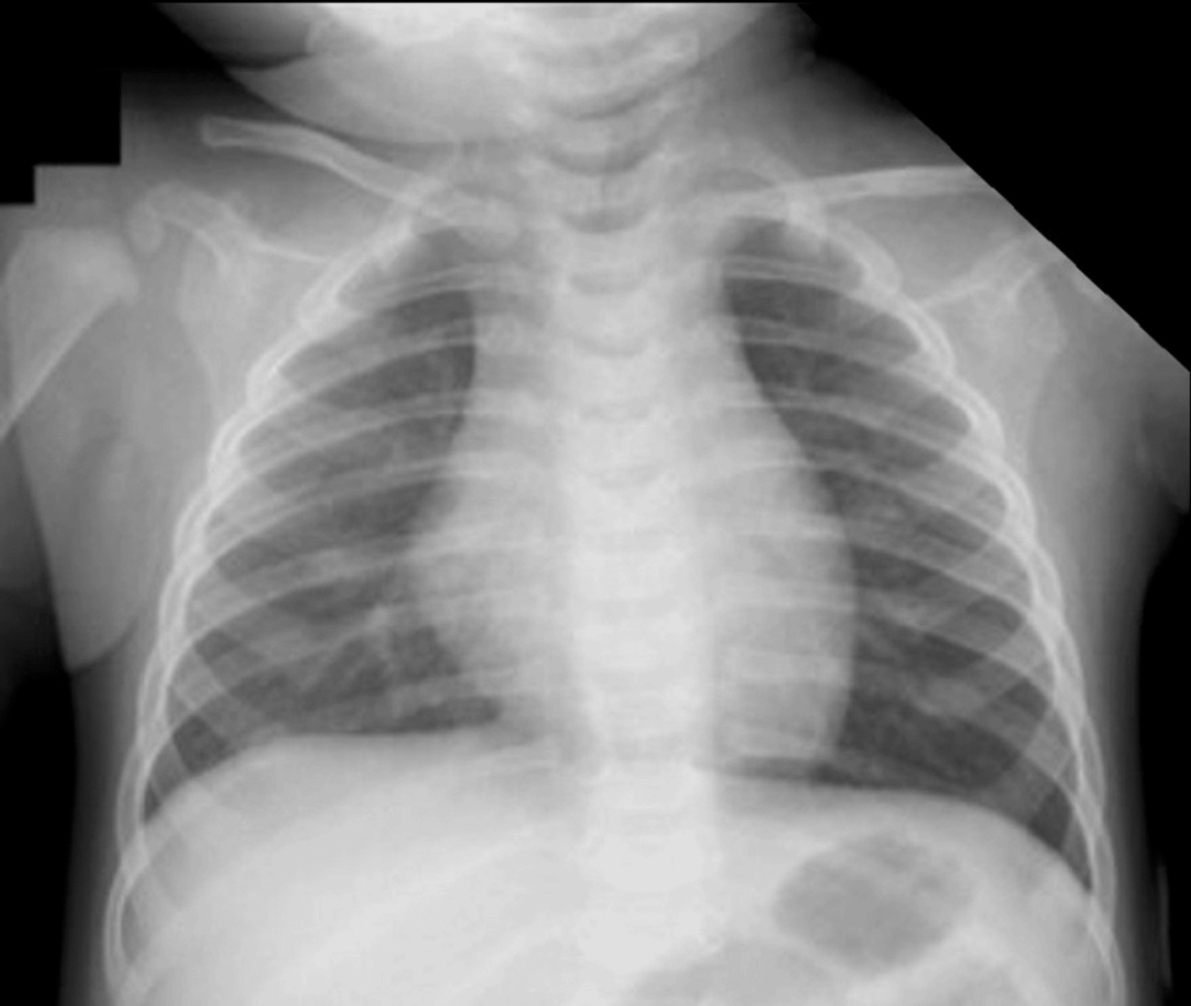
Preoperative chest X-ray The preoperative chest X-ray shows no abnormal findings in the lung field and mediastinum.
